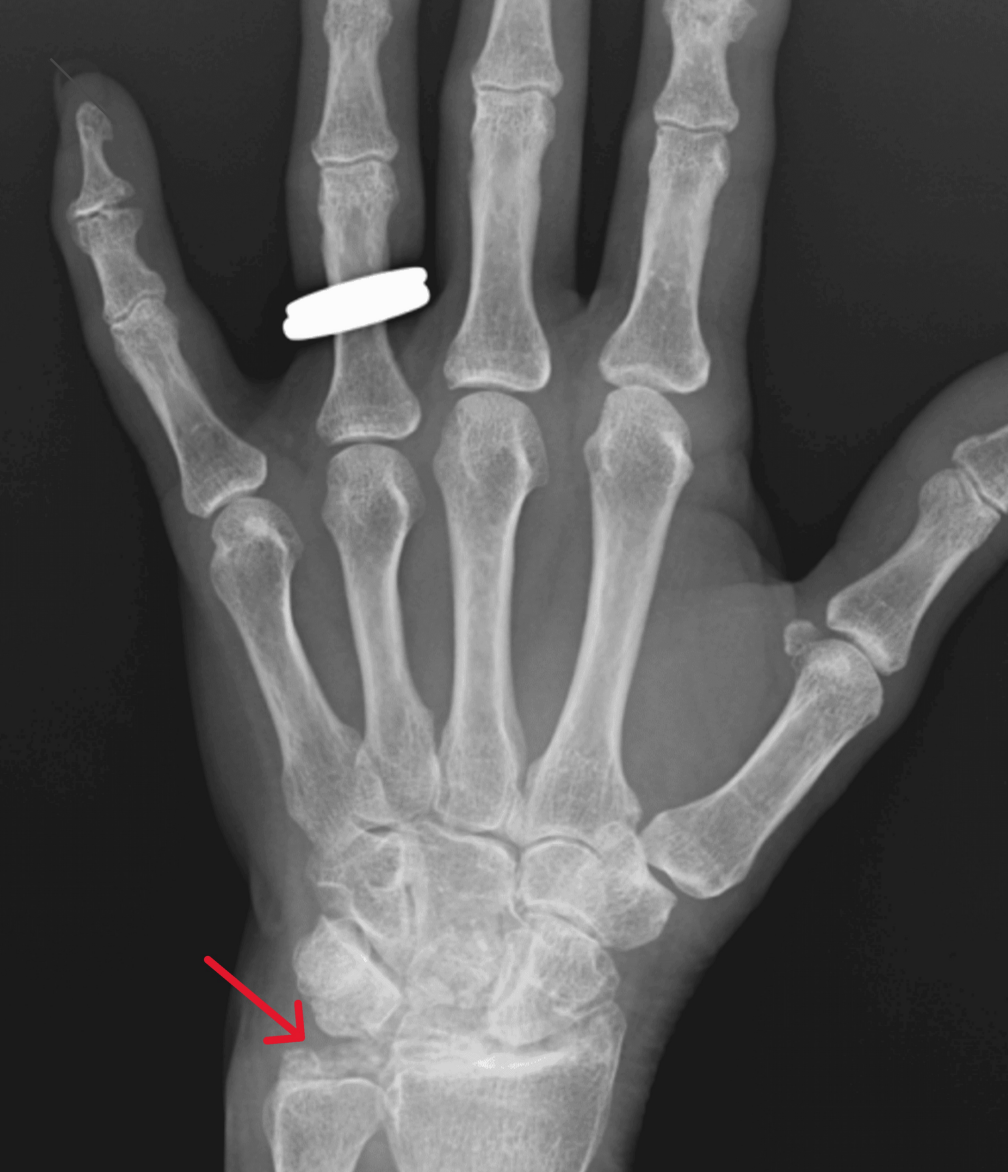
X-ray of the left hand and wrist from 2023, showing the presence of arthritic changes and evident calcifications of the triangular ligament on the left
